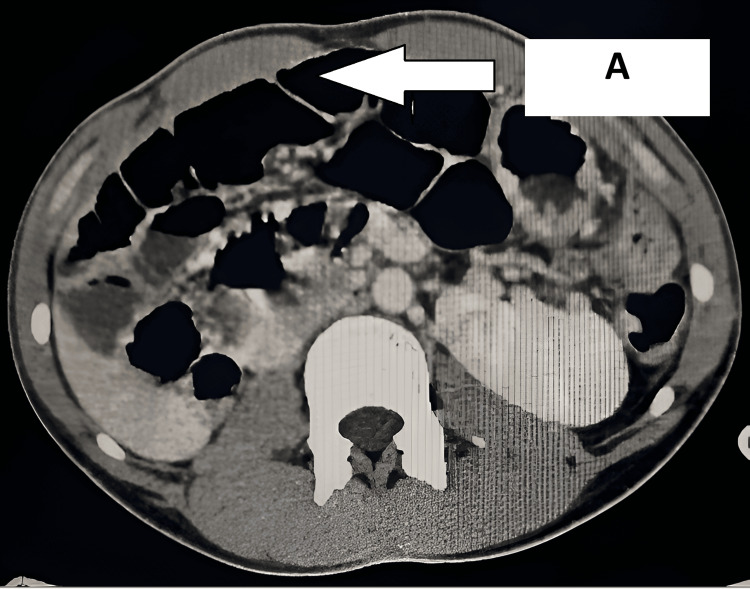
CECT of the abdomen (axial view) A: CECT of the abdomen showing a dilated bowel loop. CECT: contrast-enhanced computed tomography
